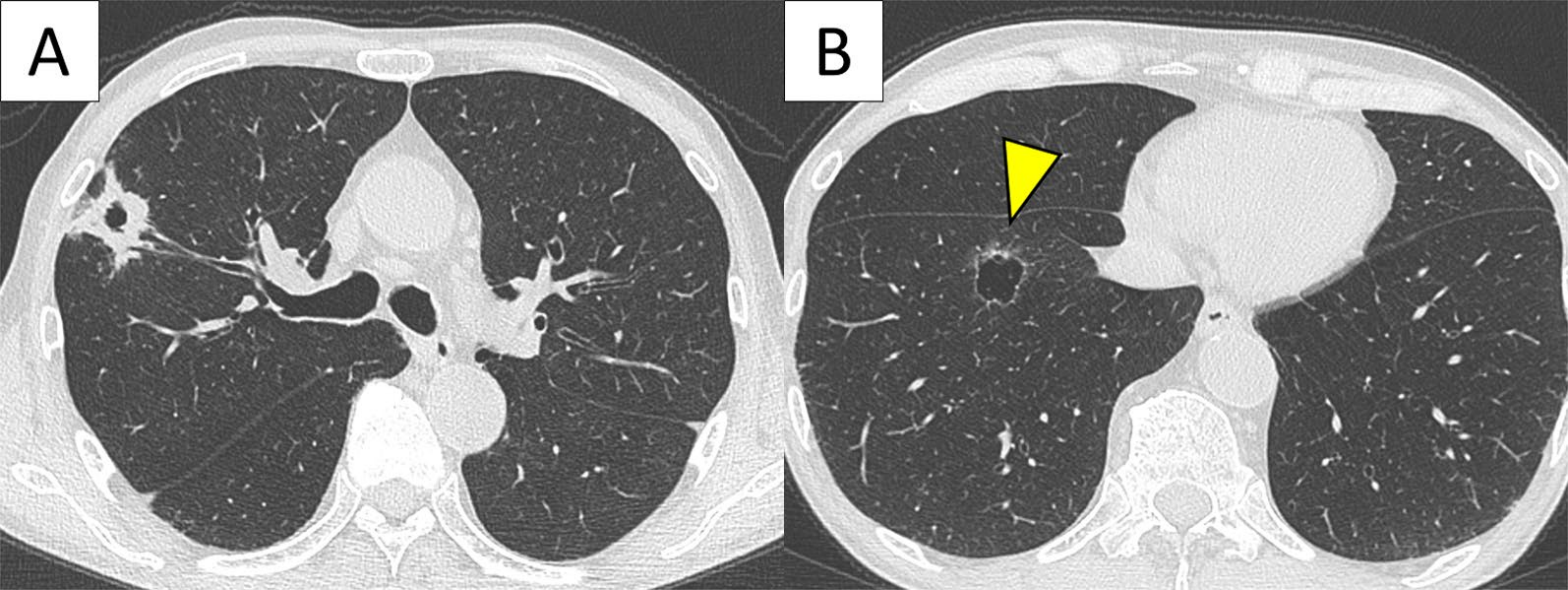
Imaging findings. a Computed tomography (CT) findings showing a 2.9 × 2.0-cm nodule on the right-upper lung. b CT scan also shows a thin-walled cavitary lesion with partly thickened areas on the right-lower lobe (arrow indicated the partly thickened cavitary wall)
